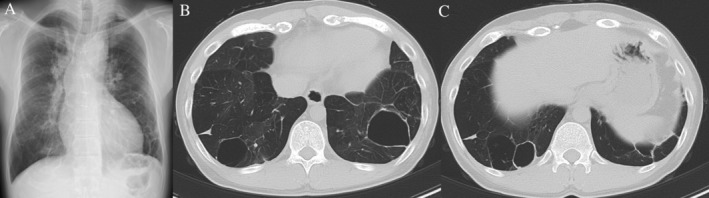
(A) Chest X‐ray at the end of treatment showed that the air‐fluid level in the left lung bulla disappeared. (B, C) Chest computed tomography (CT) at the end of treatment showed that the intrabullous fluid in both the lungs disappeared and left bulla decreased in size. Wall thickness of both bullae reduced.
